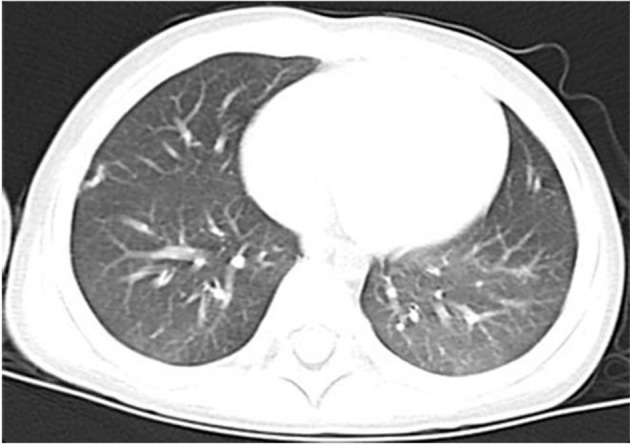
Chest CT showed significant improvement in both lungs on August 2018.
